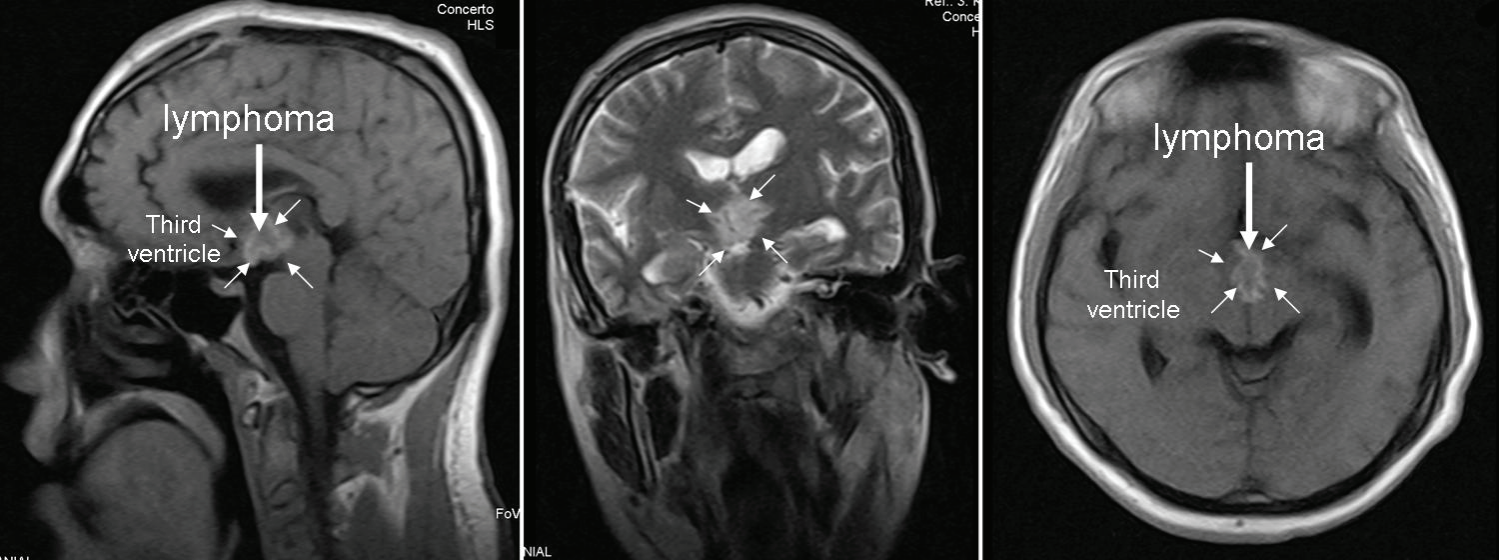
A pre-operative cranial MRI showing the primary lymphoma (arrow), which was solely located in the third ventricle (small arrows).
